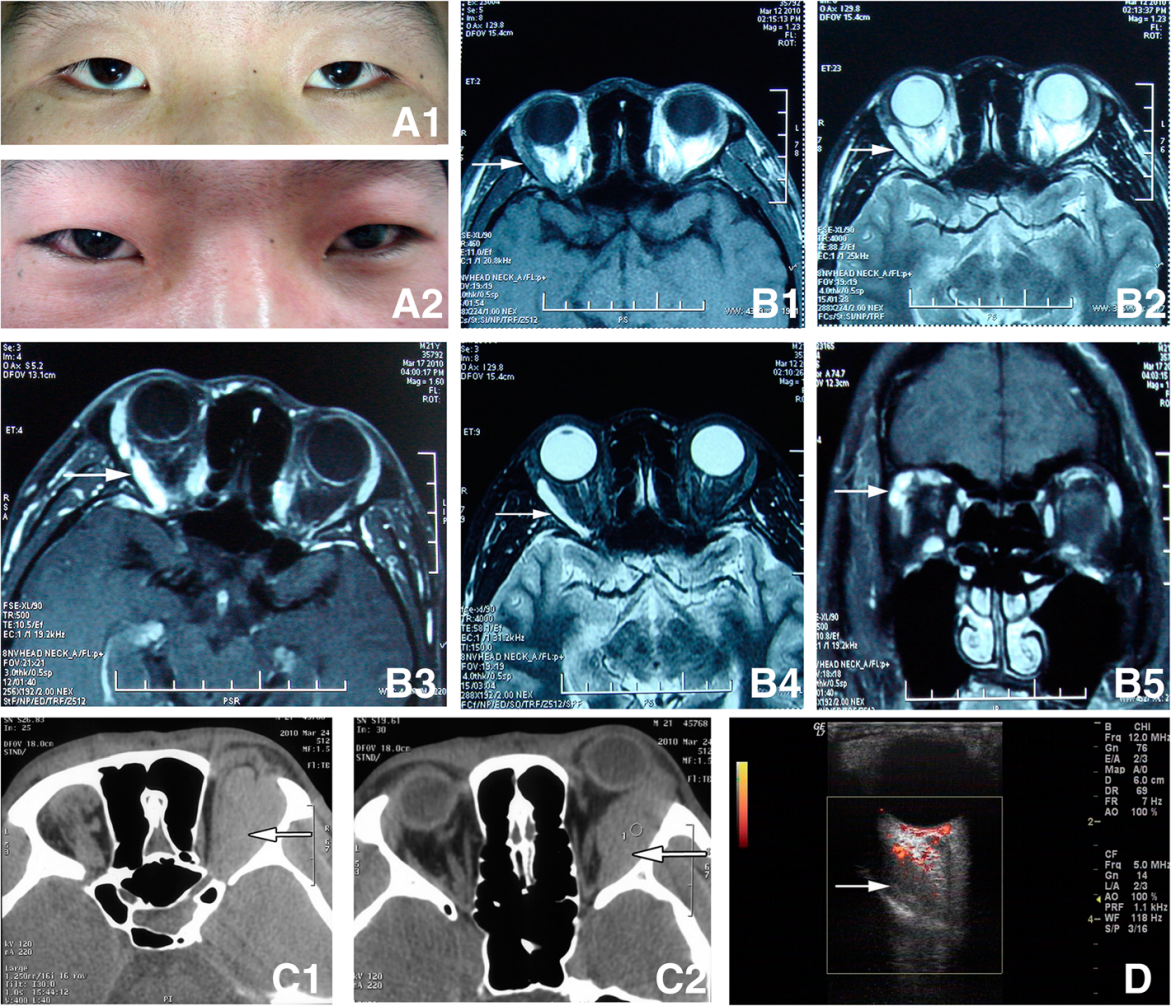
Patient with intra-orbital varicosity *A1*. Appearance when the patient was sitting, Hertel’s exophthalmometry revealed 2 mm of enophthalmos of right eye; *A2*. Appearance when the patient had bent down and bowed his head for 1 min, 4 mm of proptosis of right eye than the left; *B*. Horizontal MRI and coronal scan(supine position), showed a superotemporal ribbonlike mass in the extra-conal orbital compartment. The lesion displayed isointense on T1-weighted images (B1), hyperintense on T2-weighted images (B2), and significantly heterogenous contrast enhancement on enhanced and fat suppression MRI scan (B3–5). *C*. Axial CT scanning (prone position, the symbol of the right eye “R” was opposite to that on the MRI) showed a right superotemporal huge spindle-shaped homogenous mass pushing the eyeball to protrude and extending to the orbital apex. The superior rectus, lateral rectus and optic nerve were difficult to identify. *D*. CDFI (30 mmHg pressure was applied to the neck by the cuff of sphygmomanometer) showed a ribbonlike well defined hypoechoic mass expanded. The lesion was affecting the lateral rectus and pushed the optic nerve moving inward. There were signals of blood flow in the lesion. The arrow pointed to the lesion in Fig. 2
